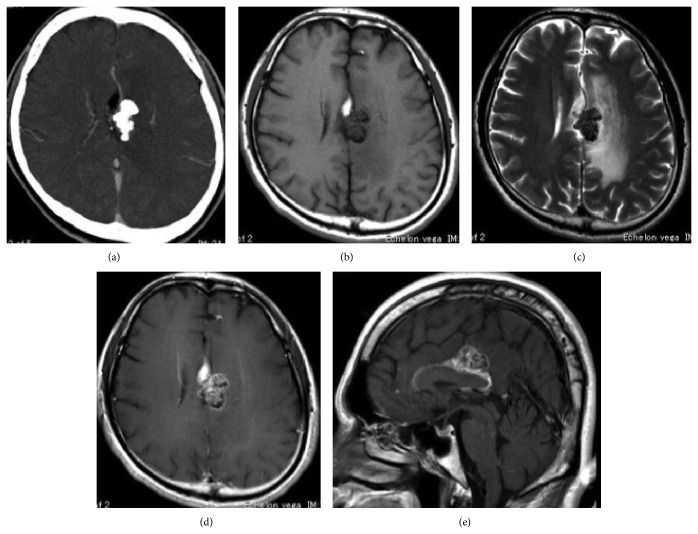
(a) Preoperative computed tomography (CT) showing calcified tumor in the interhemispheric fissure. (b, c) Preoperative magnetic resonance imaging (MRI) showing a lesion along the corpus callosum, appearing hyperintense on T1-weighted imaging (b) and isointense on T2-weighted imaging (c) associated with expanding perifocal edema. (d, e) The mass shows heterogeneous enhancement with gadolinium or axial (d) and sagittal images (e).
